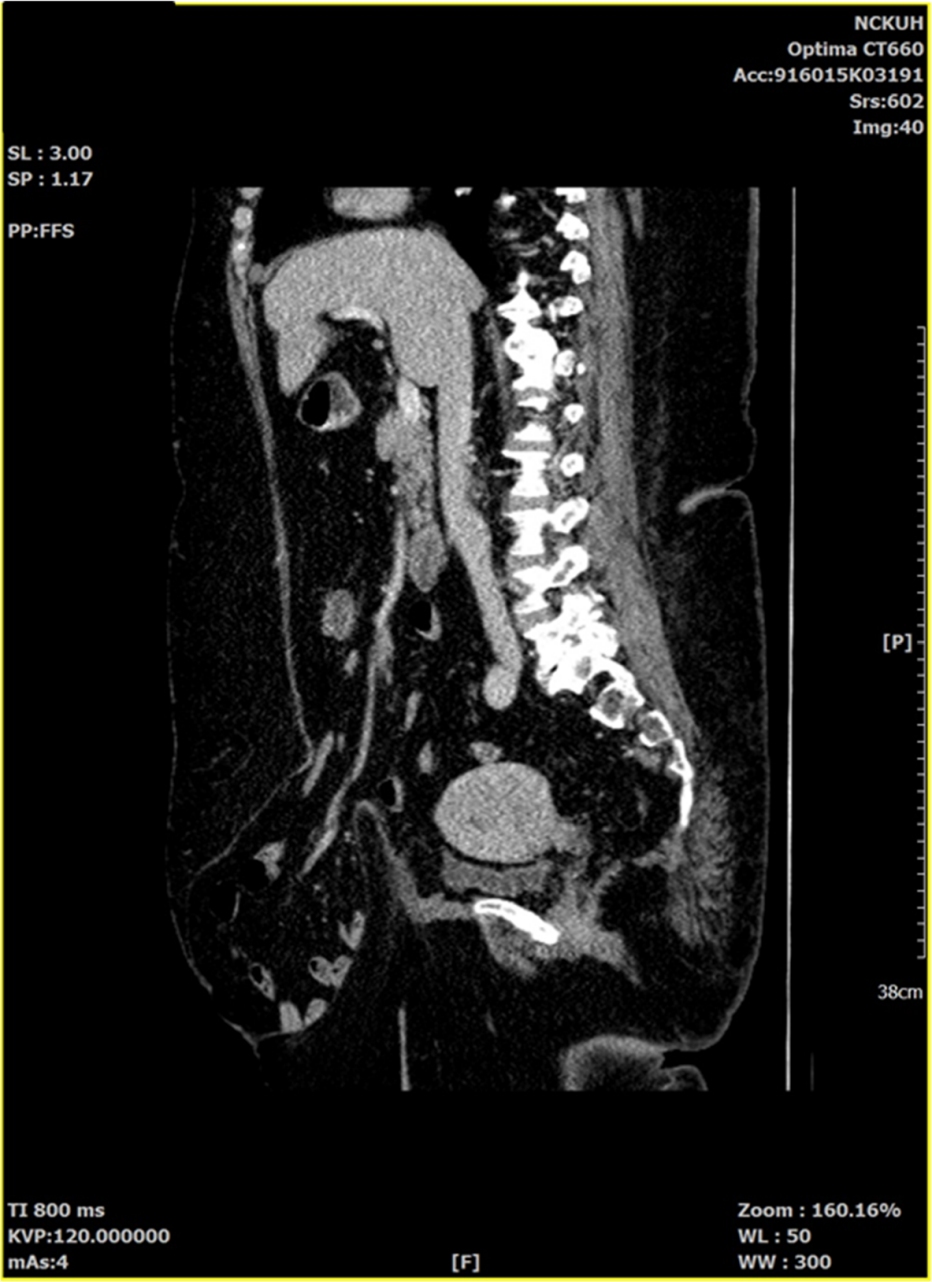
Computed tomographic image showing a large ventral hernia sac
